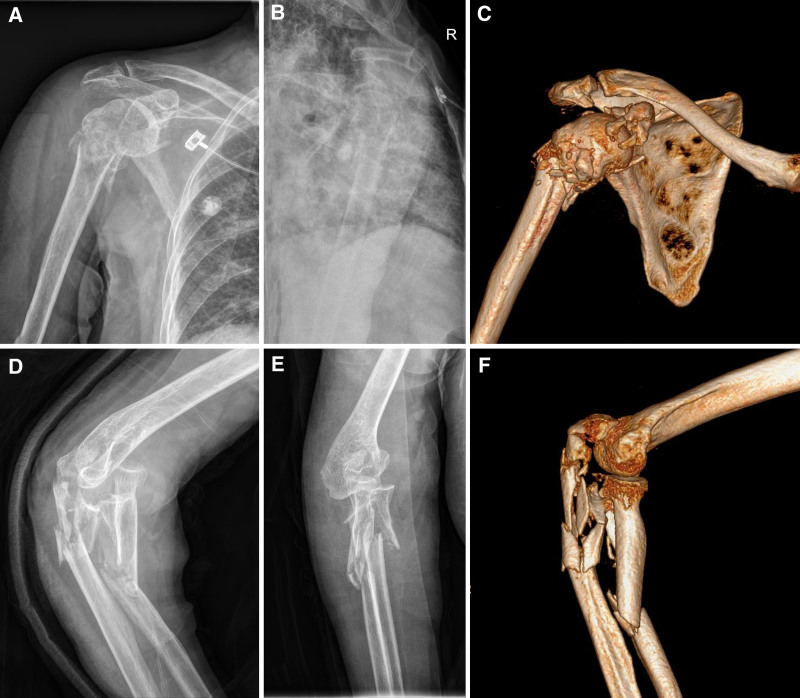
Patient’s initial pre-operative plain radiographs and computed tomography (CT). (A–C) The patient sustained comminuted intra-articular fractures of the right proximal humerus (AO/OTA fracture type C3), and fractures of the glenoid and coracoid. (D–F) An open fracture of the right proximal radius (2R2B) and comminuted intra-articular fracture of the proximal ulna (2U1C) were confirmed. AO/OTA = Arbeitsgemeinschaft für Osteosynthesefragen Foundation/Orthopaedic Trauma Association.
